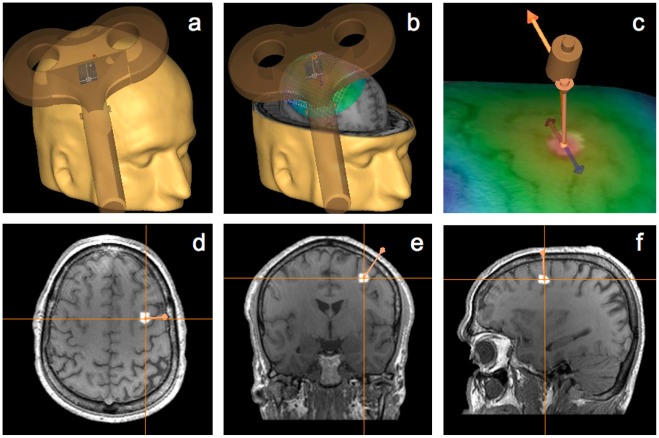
TMS targeted region, neuronavigation and coil placement. The specific location of the right FEF was identified and labeled in a three dimensional reconstruction of each participant’s MRI. The area was targeted with a 70 mm figure-of-eight TMS coil guided by a frameless stereotaxic neuronavigation system (a and b). The active TMS coil was placed flat with its center tangential to the targeted site and oriented lateral to medial and rostral to caudal orientation (c), approximately parallel to the medial portion of the central sulcus, i.e., ∼ a 45° angle with respect to the interhemispheric fissure. See axial (d), coronal (e) and sagittal (d) MRI views of the location for the TMS targeted right FEF (see Material and Methods for full details on the targeting strategy).
